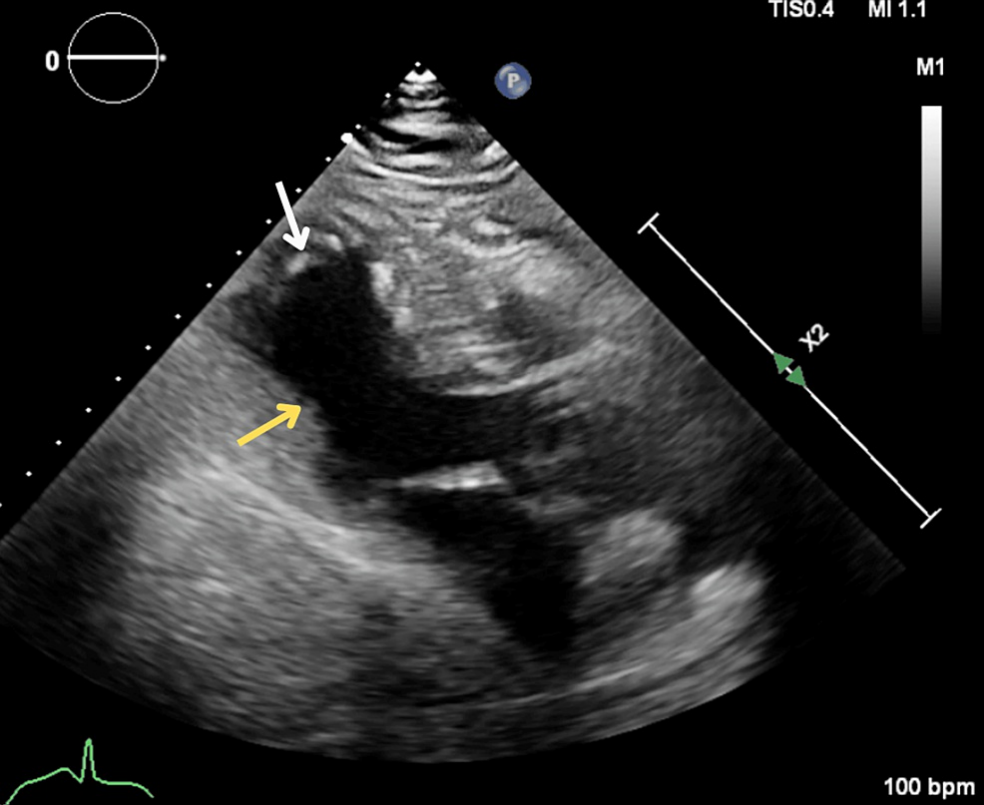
Initial echocardiogram. Parasternal long-axis view during systole, revealing concentric left ventricular hypertrophy with apical ballooning (white arrow) and preserved basal contractility (yellow arrow).
